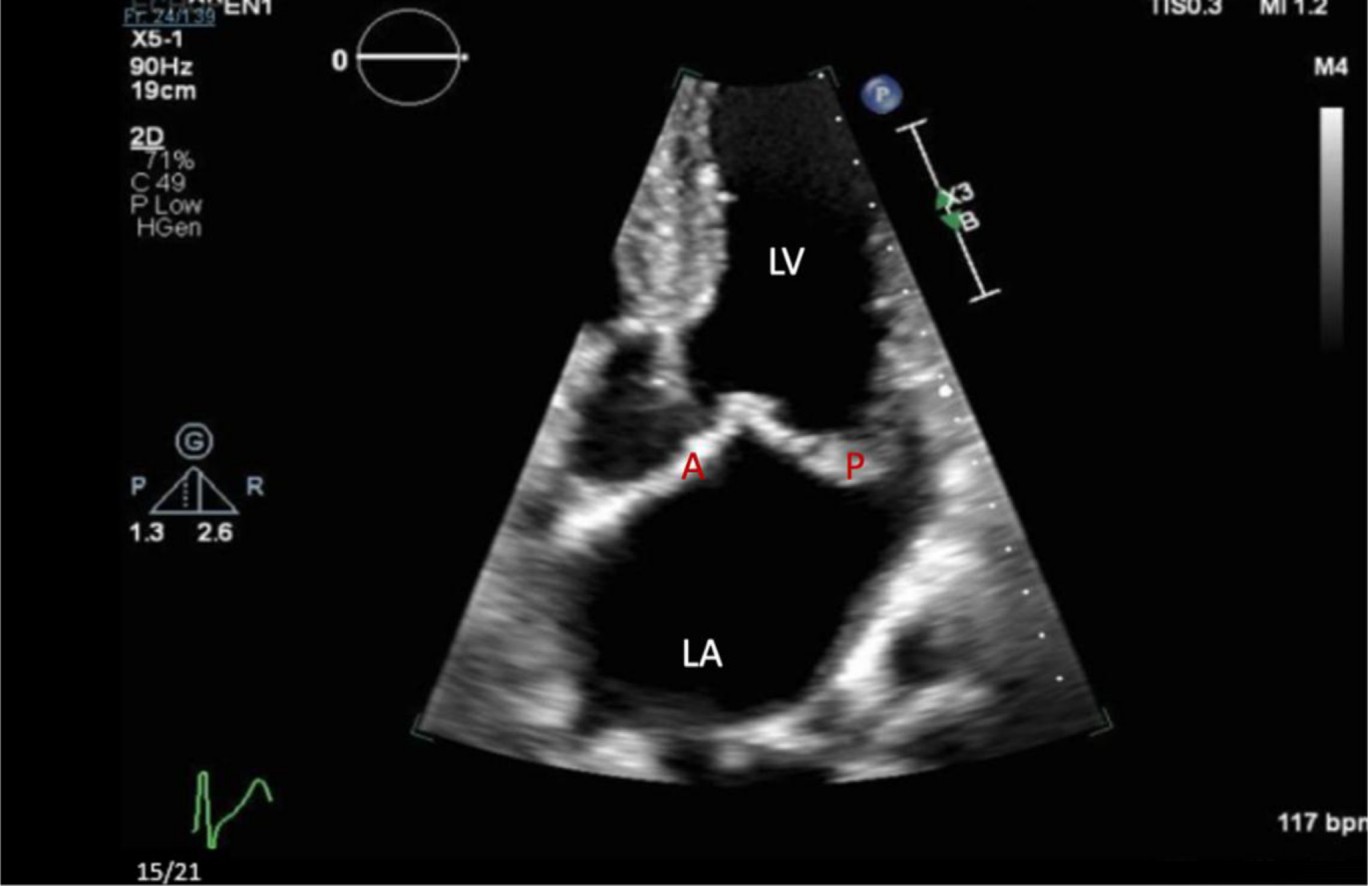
Zoomed in transthoracic echocardiogram with apical four-chamber view showing the left ventricle (LV), left atrium (LA), posterior (P), and anterior (A) mitral valve leaflets.
